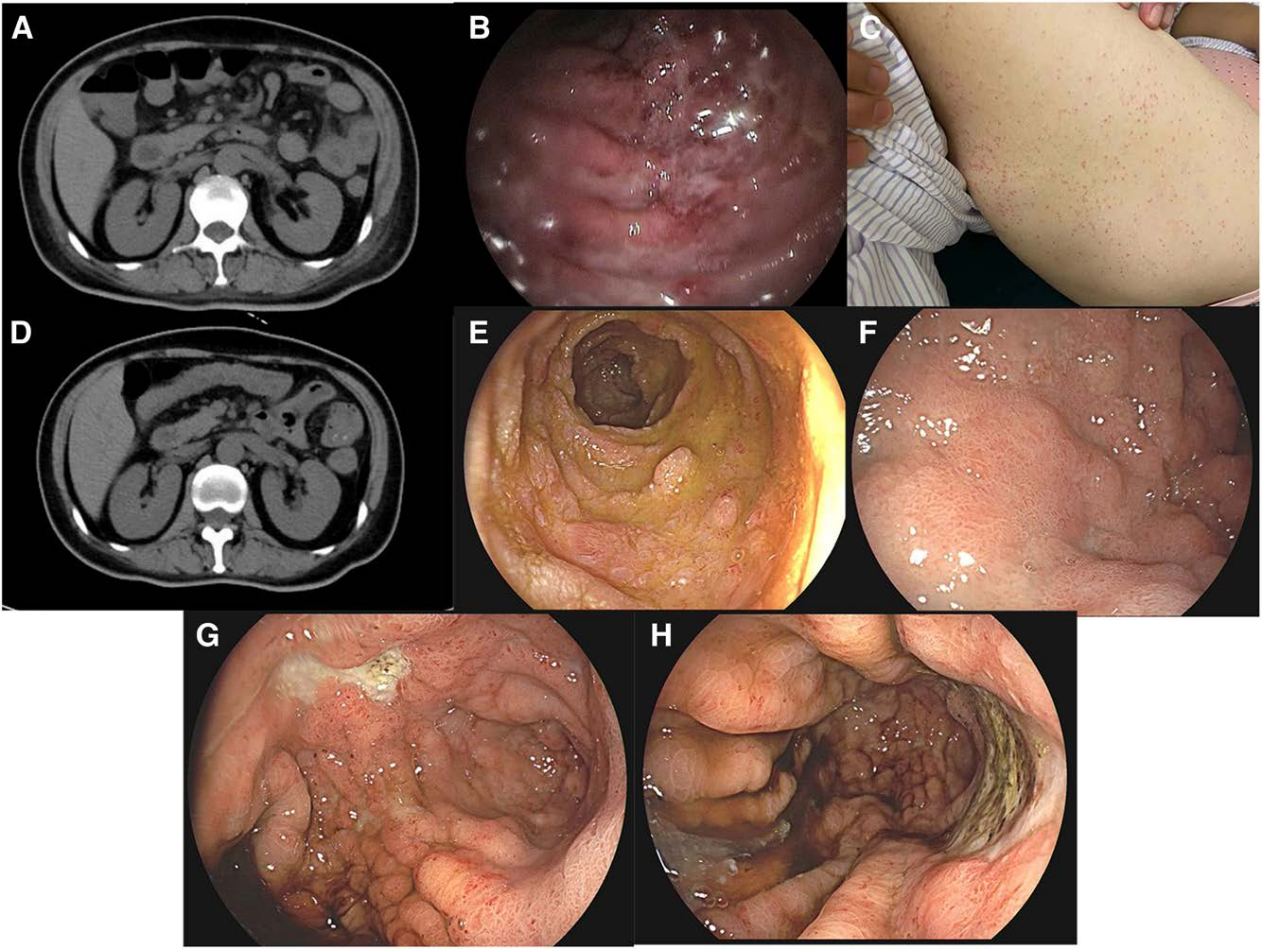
Radiologic and endoscopic imaging of the patient. (A) The initial abdominal contrast-enhanced CT revealed diffuse intestinal wall thickening; (B) the initial EGD revealed congestion, erosion, and bruising of the duodenal mucosa; (C) on the third day of hospitalization, the patient developed petechial rash on the front and inner thighs; (D) Following worsening abdominal pain, a subsequent CT scan demonstrated a notable improvement in intestinal wall edema; (E–H) subsequent EGD after exacerbation of abdominal pain showed reduced mucosal congestion but newly appeared multiple and extensive gastric ulcers. CT = computed tomography, EGD = esophagogastroduodenoscopy.
